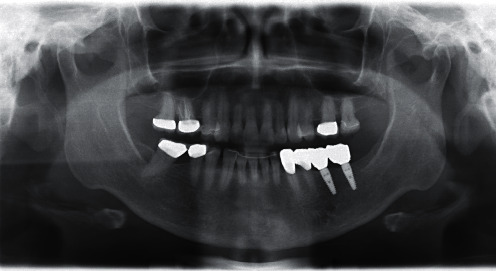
X-ray check 3 years after implant insertion.
